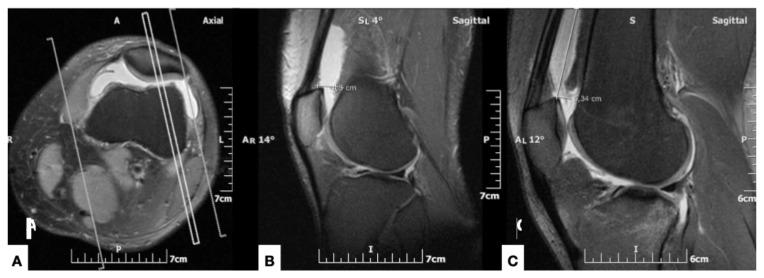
Inclusion criteria using axial (A) and sagittal ((B) and (C)) fat-suppressed proton density-weighted MR images. (A) At the level of half the patellar craniocaudal diameter, the reference for the mid-sagittal section of the patella is shown (double line). (B) Mid-sagittal section of the patella, shows the measurement of the anteroposterior diameter of the supra-patellar bursa, at the level of the upper pole of the patella. (C) At the same level as (B), in a different patient, the measurement of the craniocaudal dimension of the suprapatellar bursa is shown.
